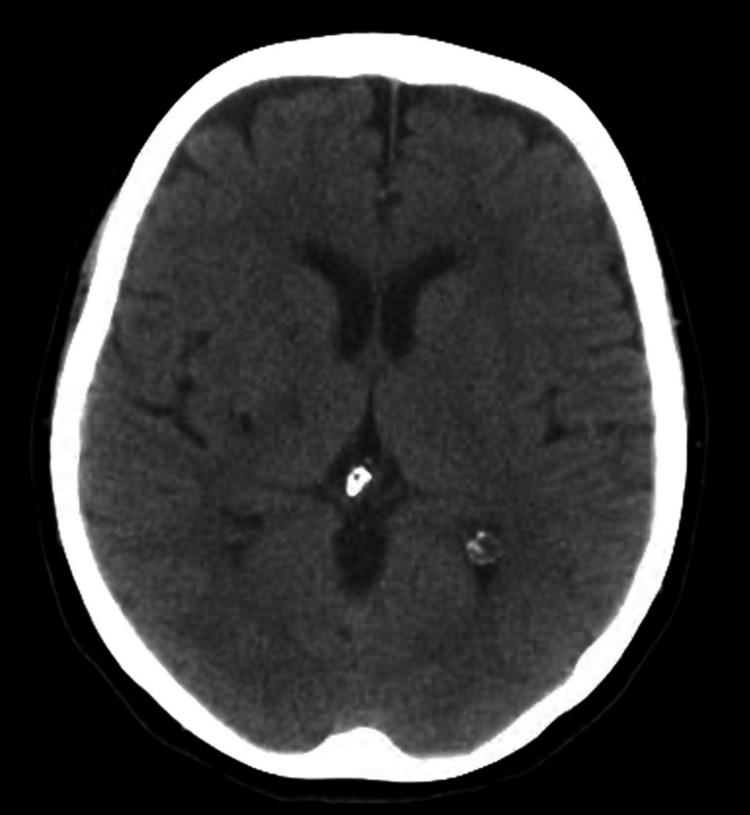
Axial view of the cerebral CT scan (noncontrast), showing no ischemic lesions CT: computed tomography
